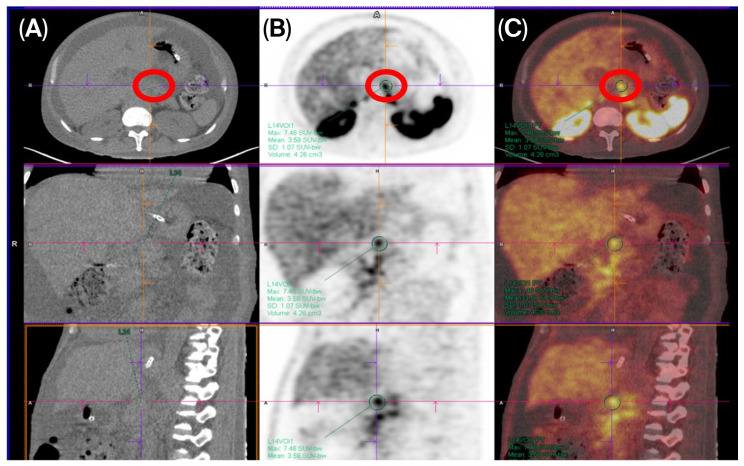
Somatostatin receptor PET/CT showing the pancreatic primary lesion. Abdominal PET/CT described a focal lesion in the pancreatic body, highlighted by the red circle. (A) Computed tomography image showing the anatomical location of the pancreatic lesion. (B) Functional PET image demonstrating increased radiotracer uptake in the corresponding region, consistent with somatostatin receptor expression. (C) Fused PET/CT image confirming the hypermetabolic lesion in the pancreatic body.
